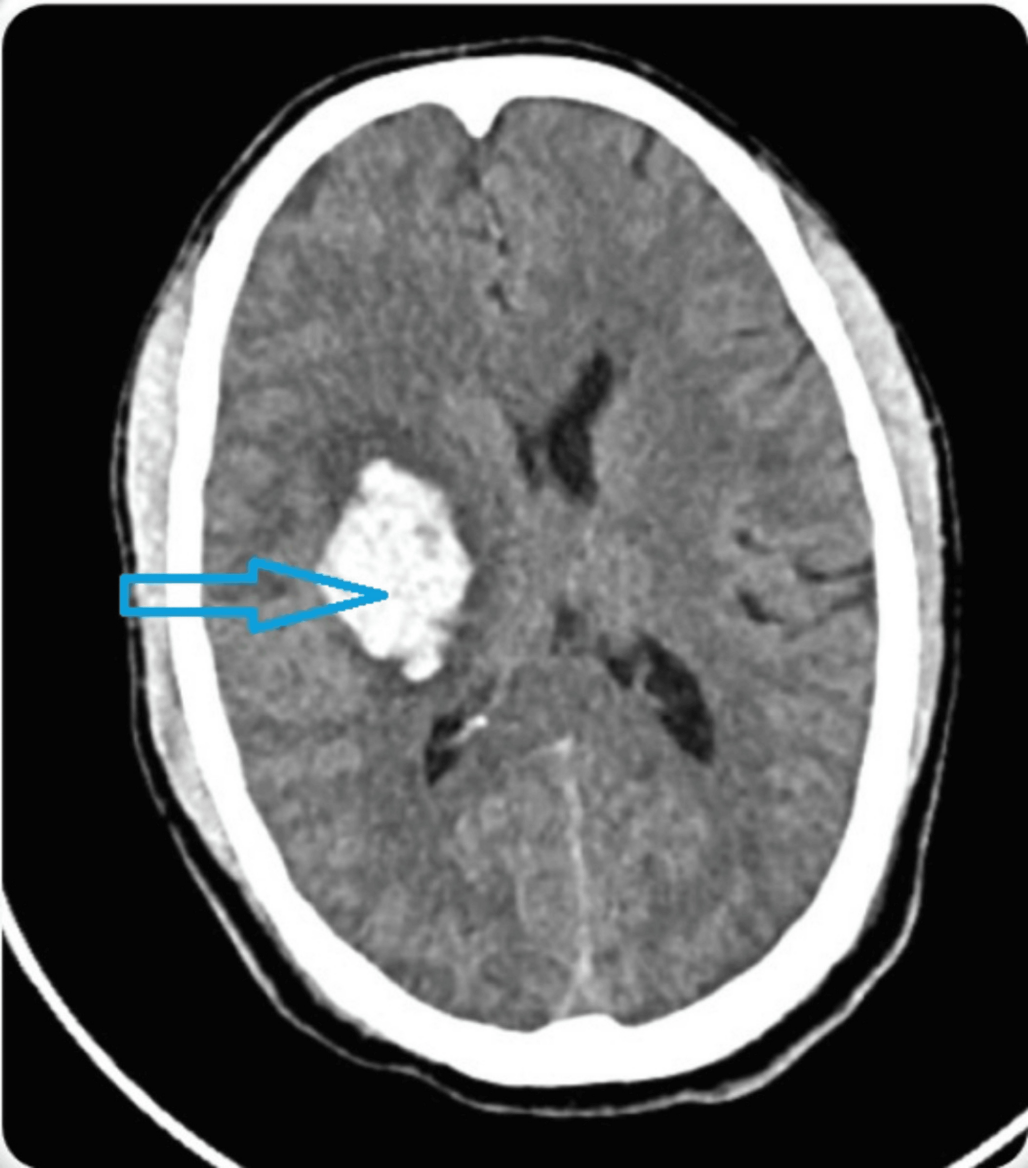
CT brain findings The blue arrow shows a well-defined supratentorial intra-axial hemorrhage measuring 3.9 x 2.9 x 3.5 cm in the right gangliocapsular region, extending to the corona radiata and centrum semiovale, with perifocal edema and midline shift CT: computed tomography
